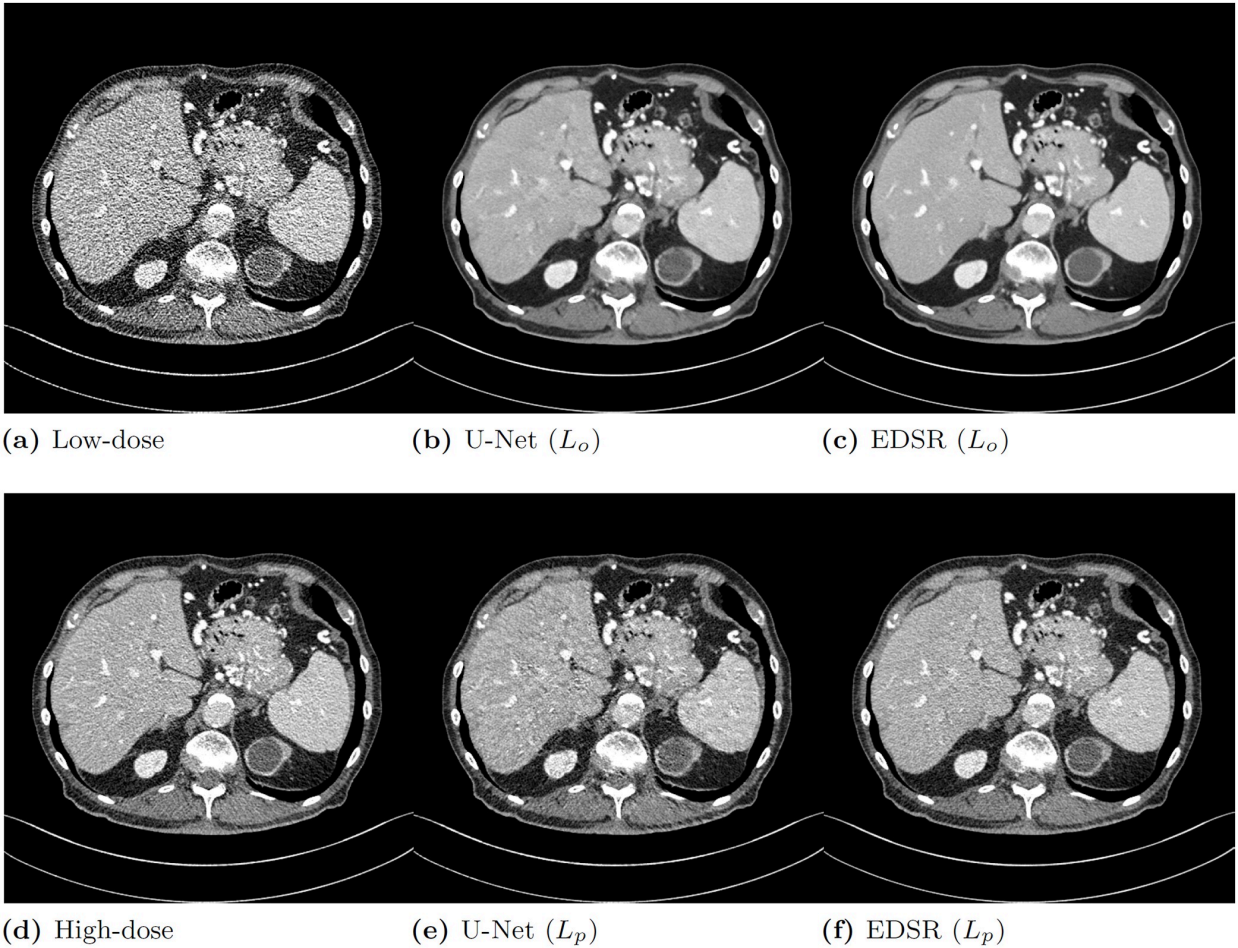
Representative examples of (a) the original low-dose computed tomography (CT) image, (d) normal-dose reference image, and denoised CT images from U-Net and EDSR optimized for objective loss (*L*_*o*_) (b) and (c) and perceptual loss (*L*_*p*_) (e) and (f).
